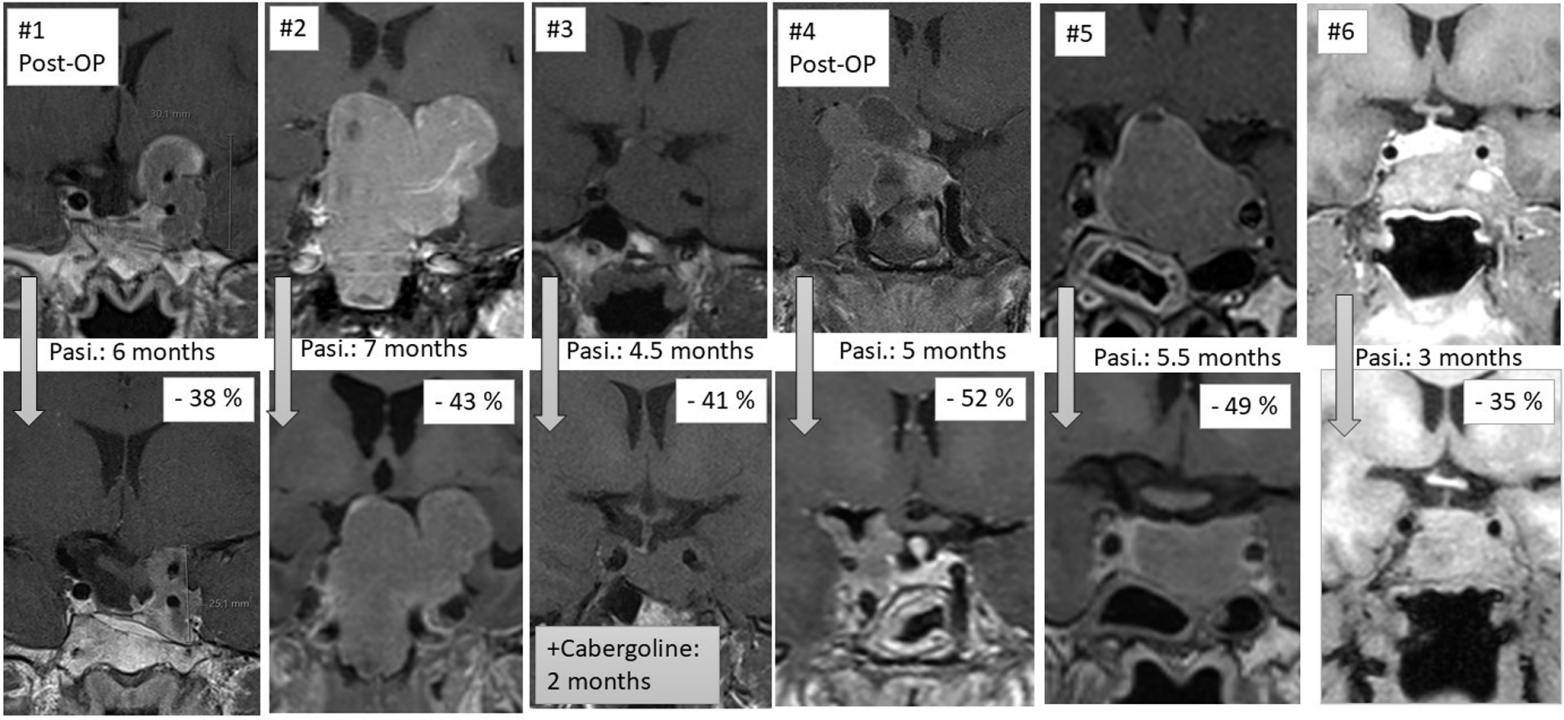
MRI before pasireotide treatment (upper row) and after 3–7 months. Tumor volume reduction as assessed by ellipsoid formula (lower row). MRI with T1-weighted, contrast enhanced series, except for #3 without contrast enhancement
